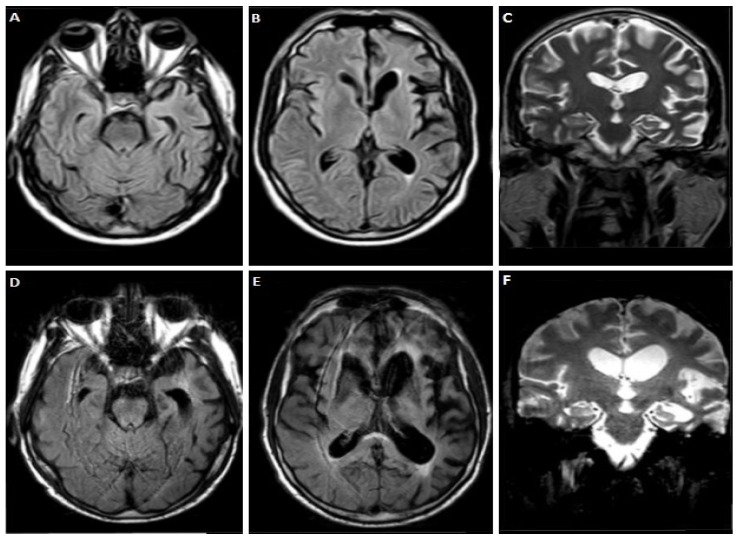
Brain MRI of case 2 at age 57 (A–C) and 60 (D–F) showing some degree of cortical atrophy in frontal and temporal lobes was observed with left predominance that deteriorated significantly three years later.
